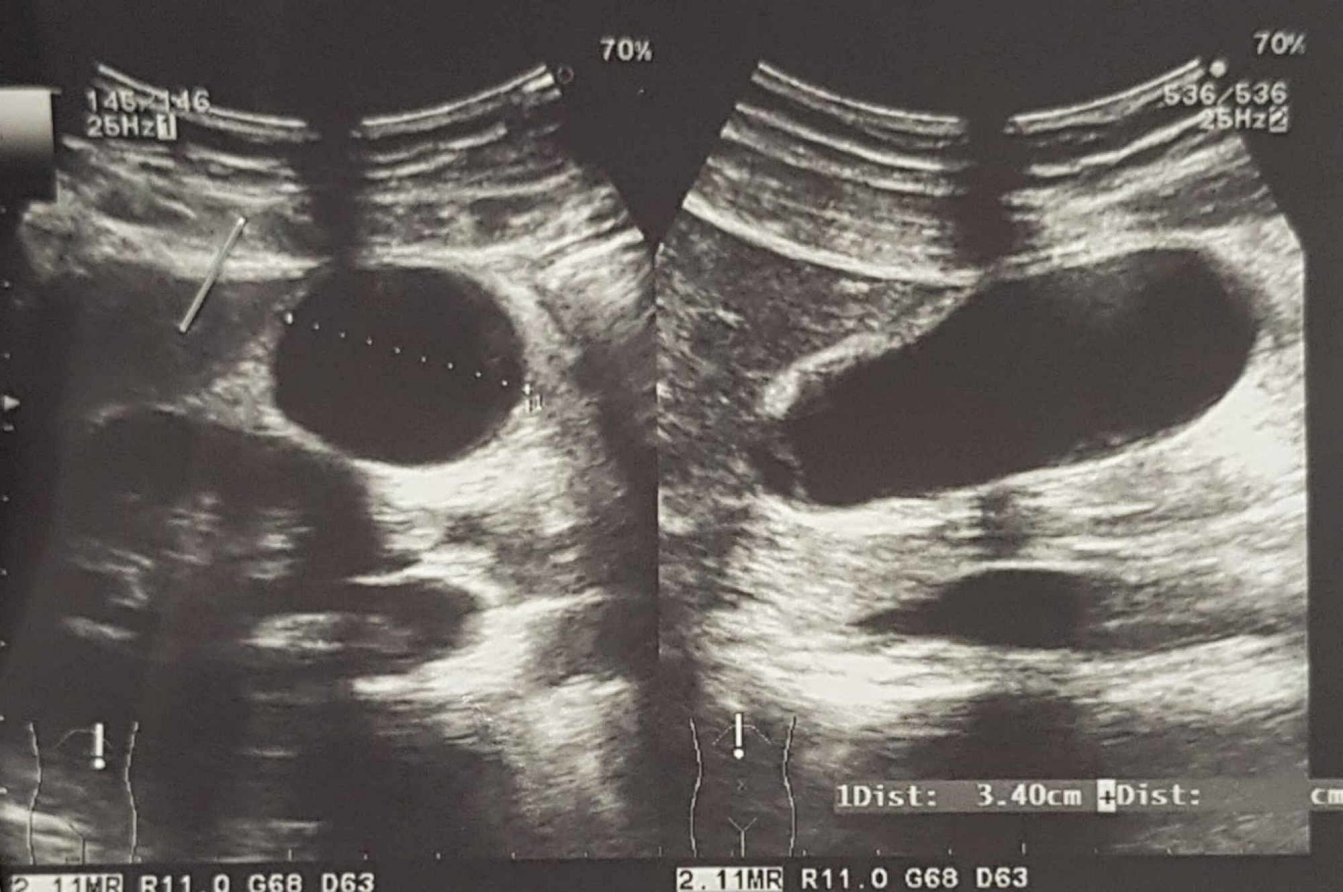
Abdominal ultrasonography The ultrasonography revealed an abnormally enlarged gallbladder without gallstones and a slightly diffuse thickened gallbladder wall
